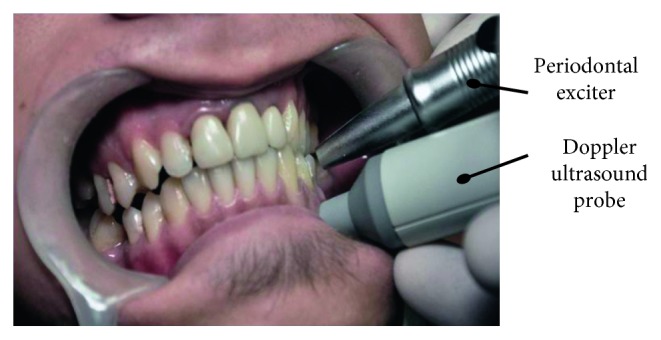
Positioning of the Periotest and of the Doppler ultrasound sensor towards an investigated tooth.
